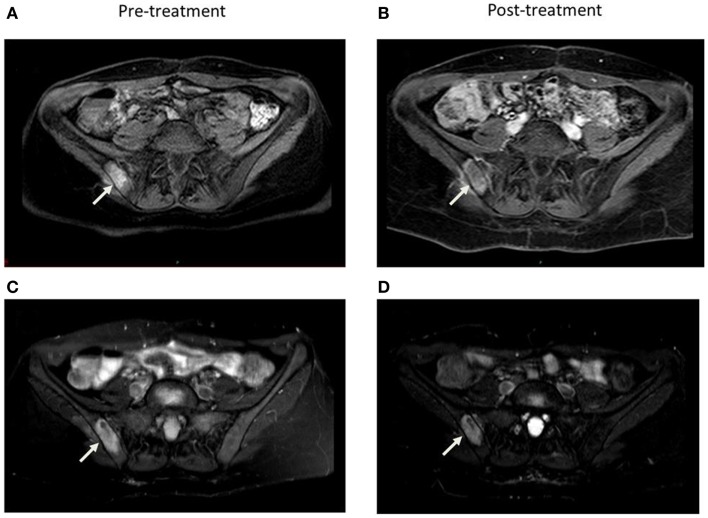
Comparison of axial 1 month pre and 5 months post-treatment contrast enhanced (A, B) and STIR (C, D) MRI images demonstrate decrease in vascularity and size of the right sacroiliac joint lesion.
